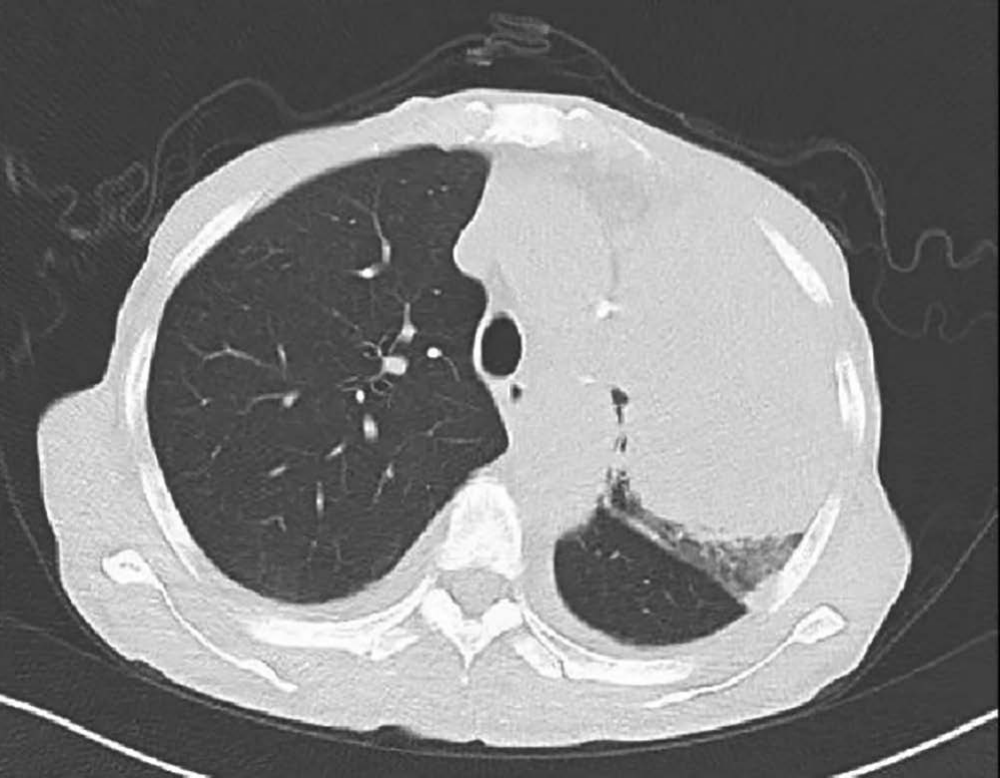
Initial chest computed tomography (CT) revealing a left upper lobe mass with bronchial obstruction.
